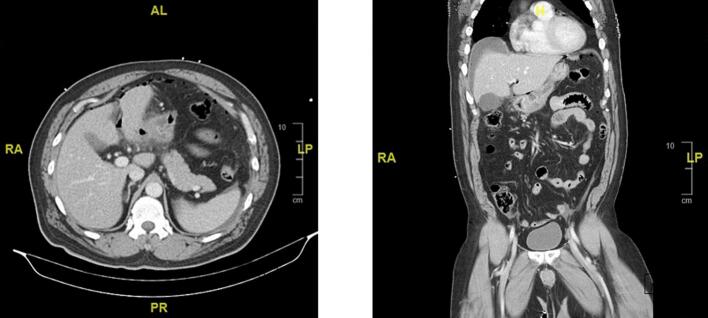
Computed Tomography showing diffuse wall thickening of the distal stomach, with associated perigastric fat standing, free fluid, and pneumoperitoneum.
